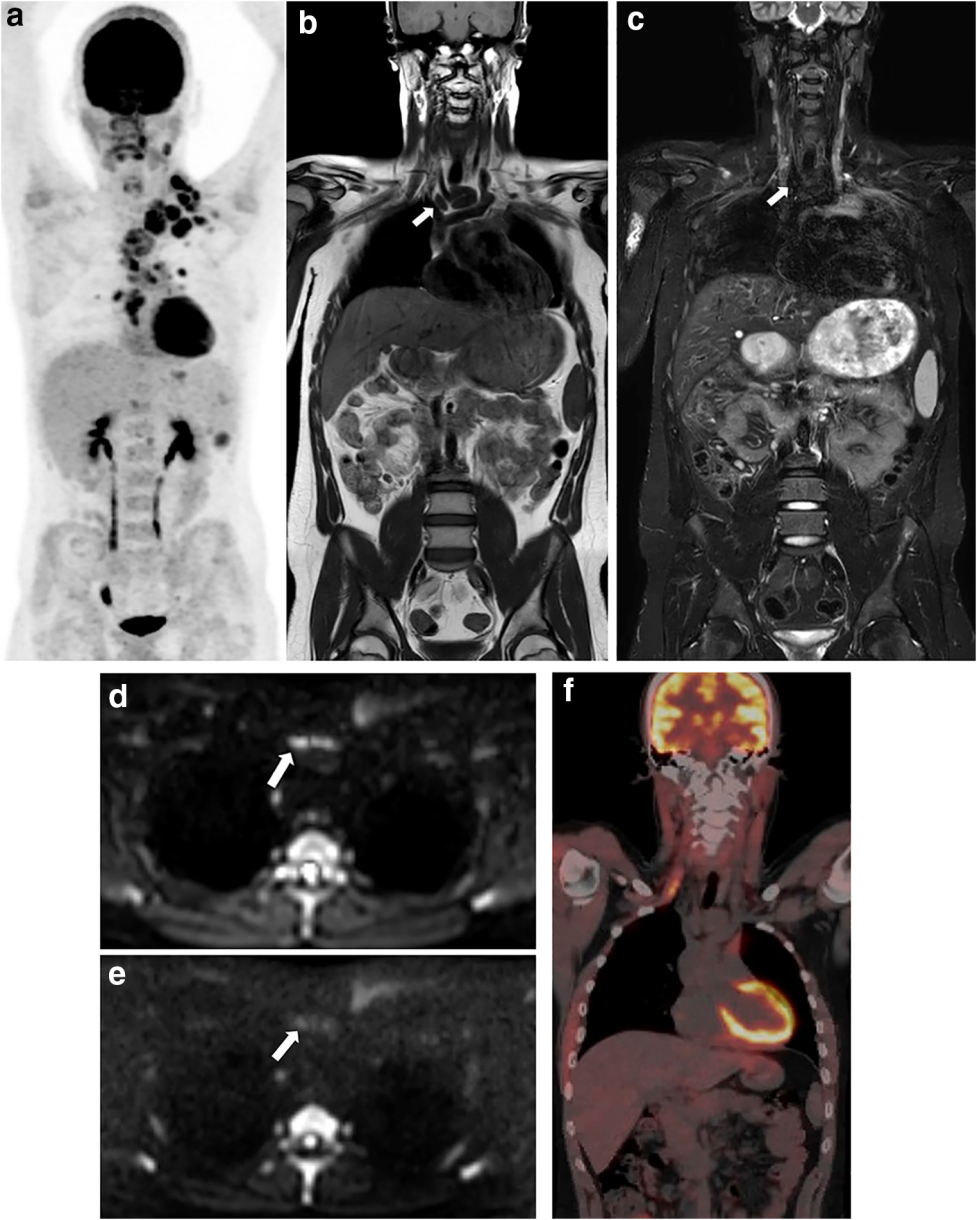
Example of end of treatment response evaluation (restaging) of a 15-year-old boy showing a fibrotic rest lesion without diffusion restriction at DWI and no disease presence at [^18^F]FDG-PET/CT. At staging, disease presence was found in the cervical, mediastinal, pulmonary hilar and para-aortic lymph node stations as well as in the spleen and right lung, indicating stage IV disease. Coronal maximum intensity projection (MIP) of the [^18^F]FDG-PET/CT (a) at staging demonstrates the affected (lymph node) stations. Coronal T1-weighted (b) and T2-weighted (c) MRI show a rest lesion at restaging (arrows). At both T1-weighted and T2-weighted MRI, low signal intensity is seen, and axial DWI (b100 (d) and b800 (e)) reveals no diffusion restriction. At axial [^18^F]FDG-PET/CT (f), no elevated [^18^F]FDG uptake is seen in the rest lesion as well indicating a complete response
